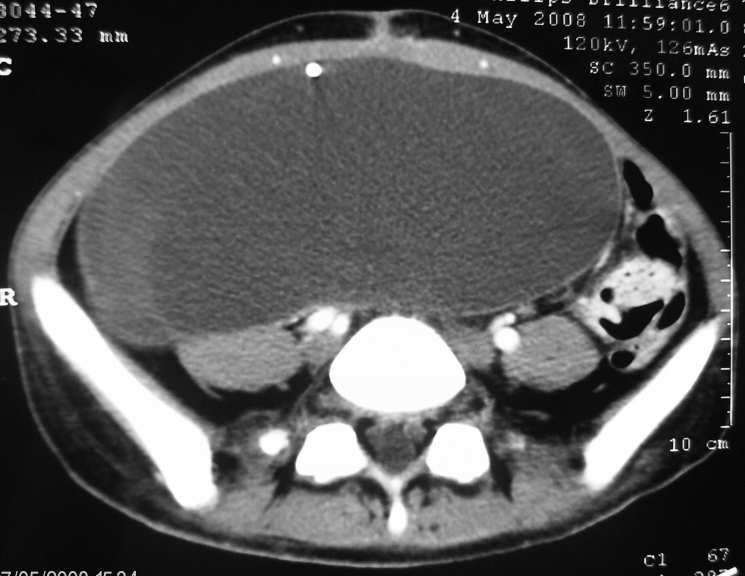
Abdominal CT. Gyant CSF pseudocyst
